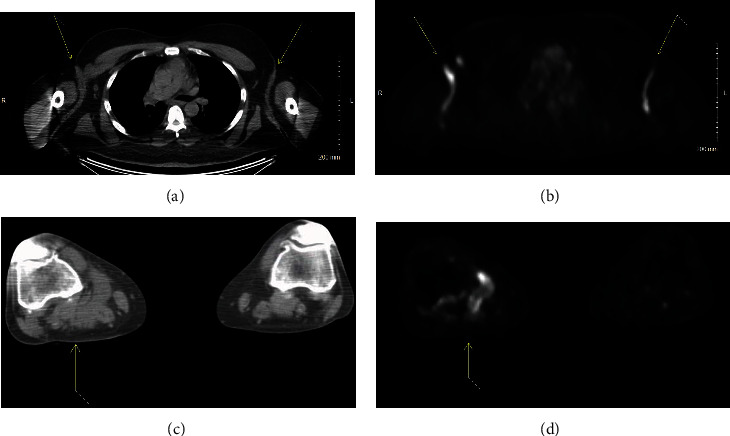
PET/CT imaging prior to starting radiotherapy. Axial CT of patient axilla (arrows) (a) and corresponding PET image (b). Axial CT of patient's right popliteal mass (arrow) (c) and corresponding PET image (d).
